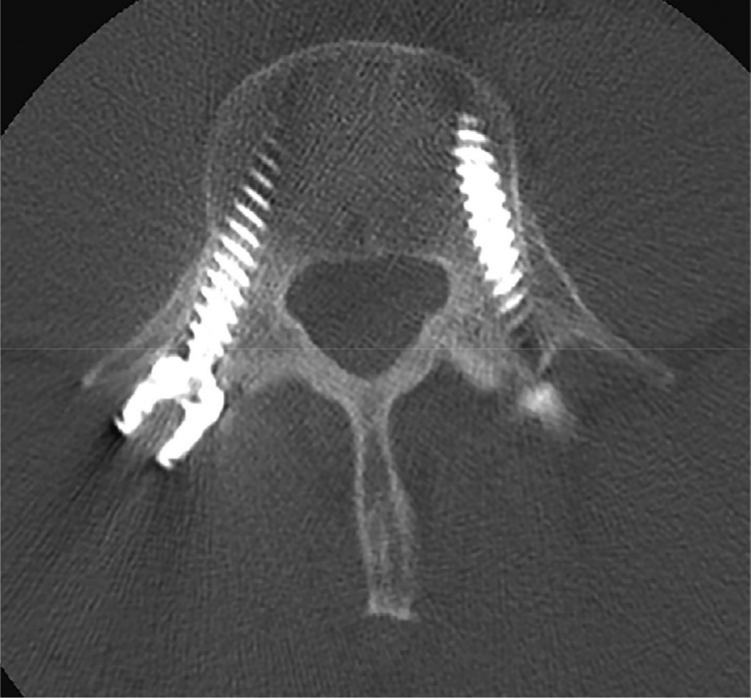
Axial postsurgical CT image showing bilateral transpedicular screws in place.
